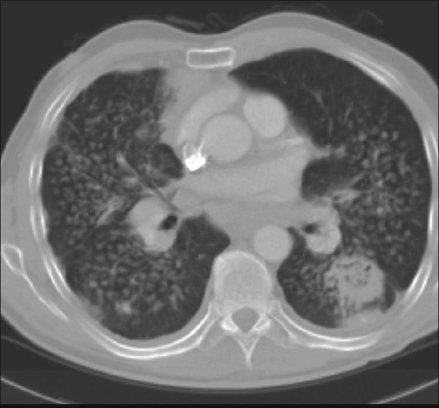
Contrast-enhanced CT (Lung window settings)
